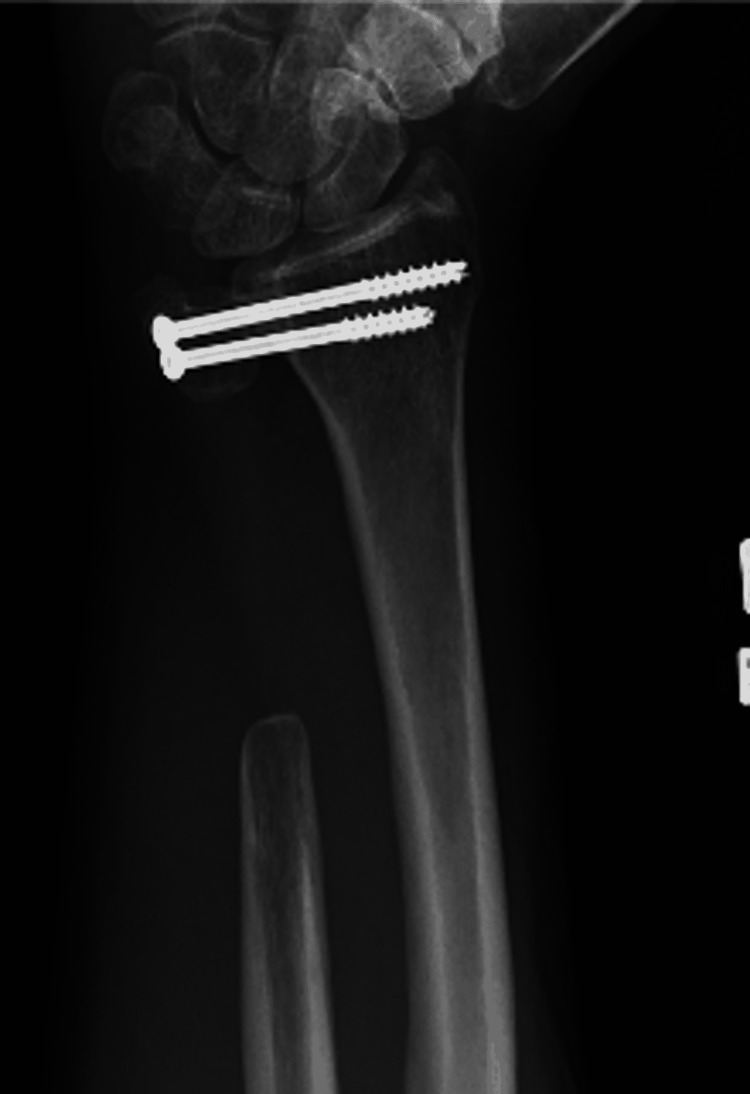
X-ray of the right forearm taken two years after the initial pre-operative X-ray. Shows prior resection of the right distal ulna with two intact metallic screws spanning the distal radioulnar joint. Overall stable postoperative changes with no findings to suggest recurrent disease.
